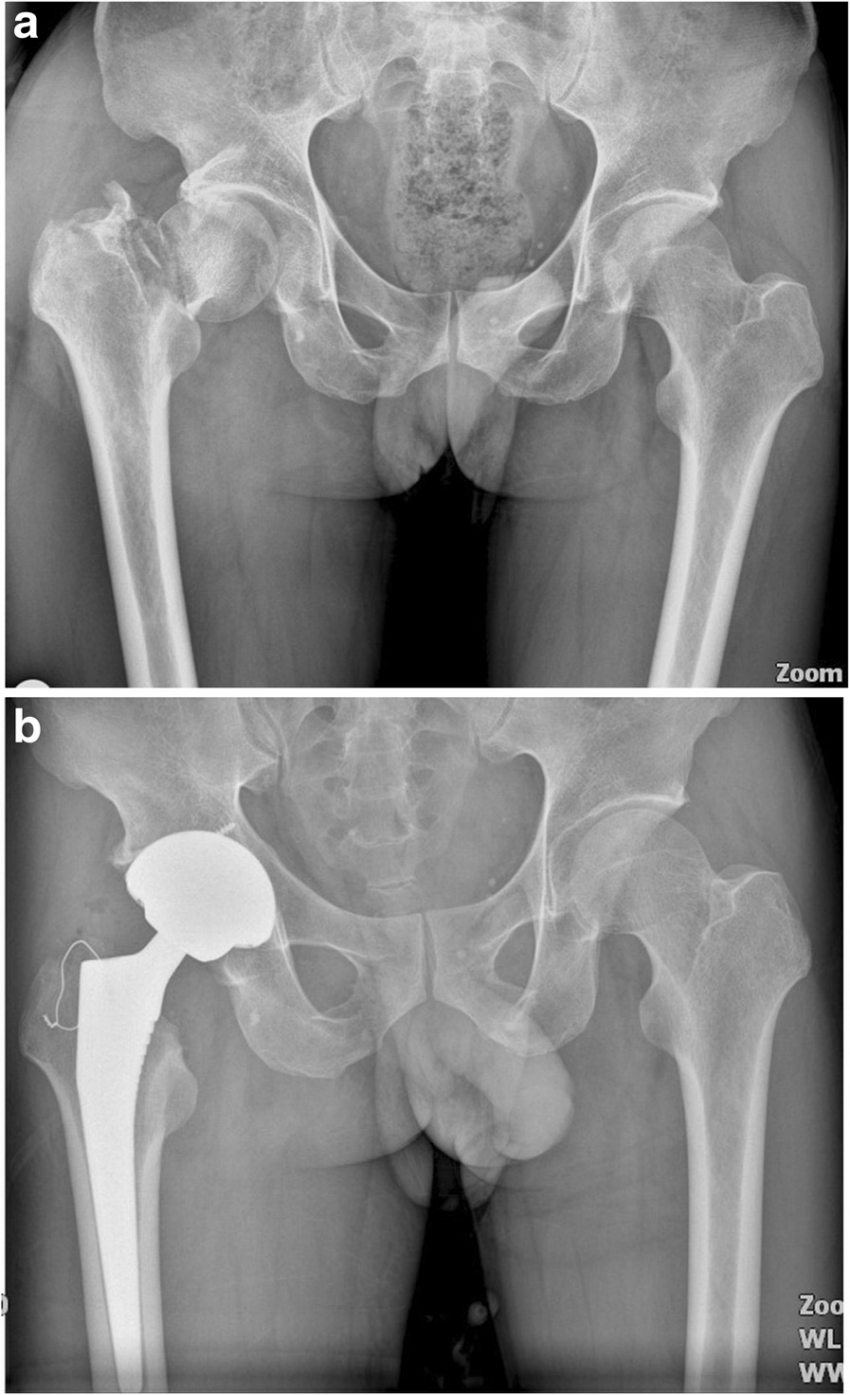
a A preoperative radiograph of the right hip of a 75-year-old man who had femoral neck fracture. b The patient had an intra-operative fracture and was treated by cerclage wire. A radiography at 42 months showed fracture healed without further complication and conventional cementless femoral stem solid fixation in a satisfactory position
